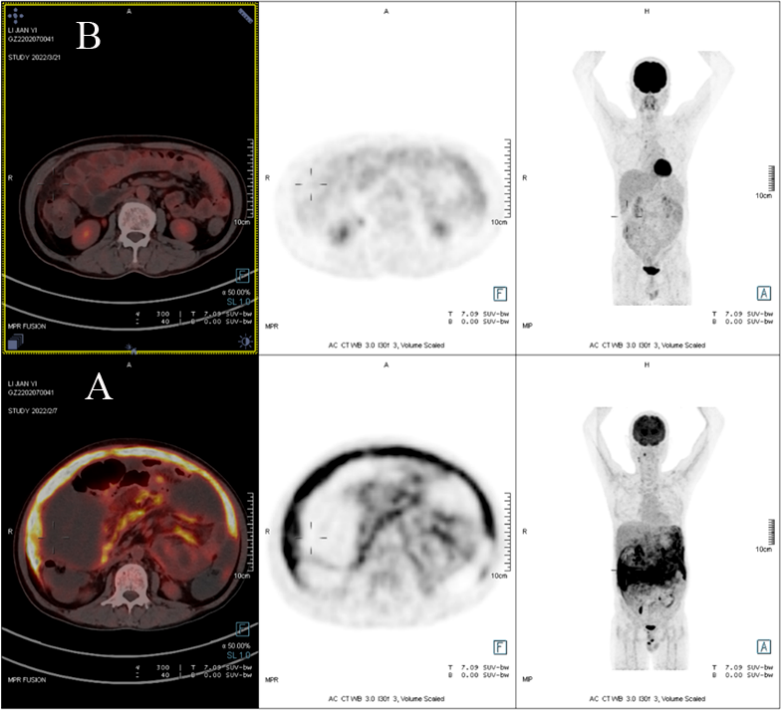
(A) PET scan demonstrating diffuse peritoneal areas of high glucose uptake before treatment. (B) PET/CT scan revealing the decline in glucose metabolism throughout the peritoneal region after treatment.
